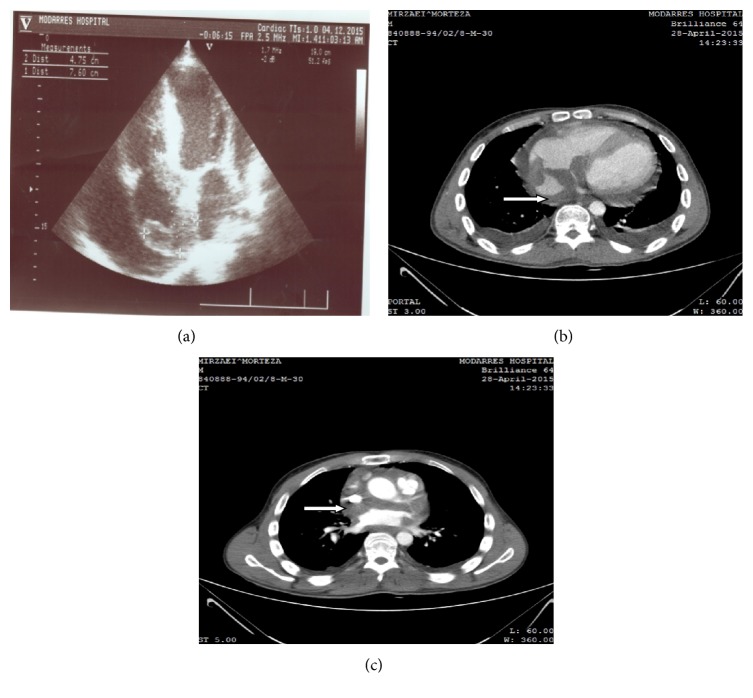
(a) TTE of patient which showed a large echogenic mass (76 mm*∗*47 mm) in the right atrium which extended toward atrioventricular junction and septal leaflet of tricuspid valve and pericardial effusion around LV, RV, and RA with no tamponade physiology. (b and c) Spiral CT-scan of the chest with and without IV contrast which showed an infiltrative circumferential mass lesion (*white arrow*) involving the right walls of the right atrium and also interatrial septum with extension to the upper part of interventricular septum.
